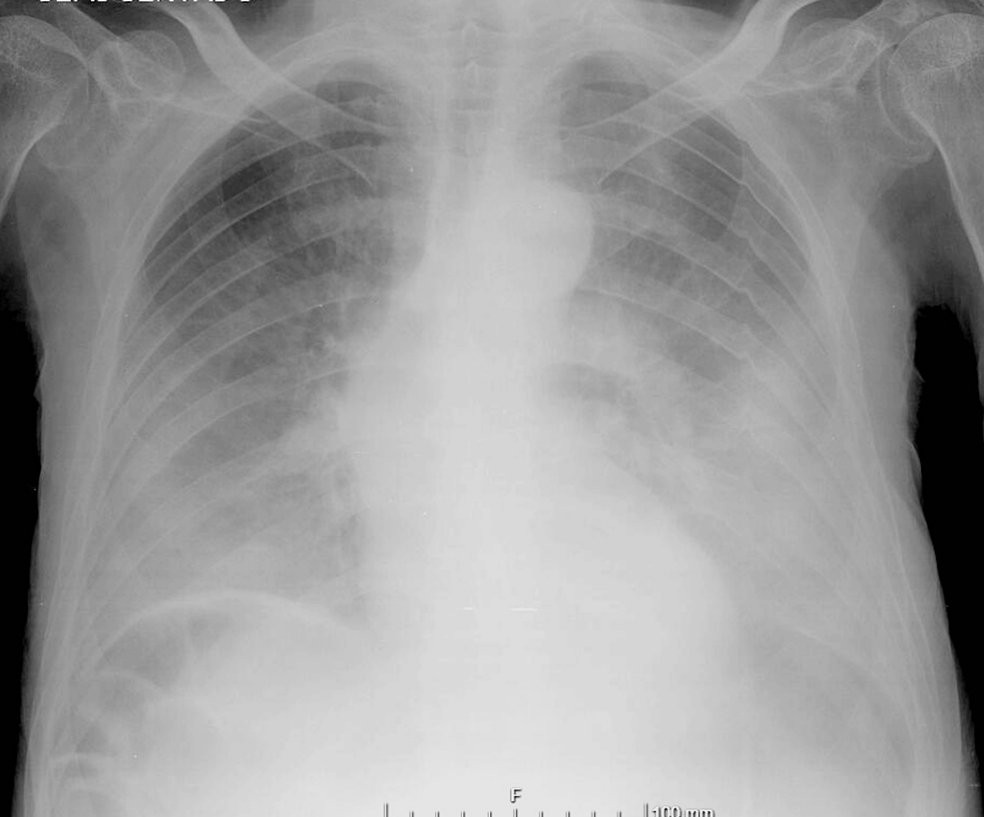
Chest X-ray on readmission
